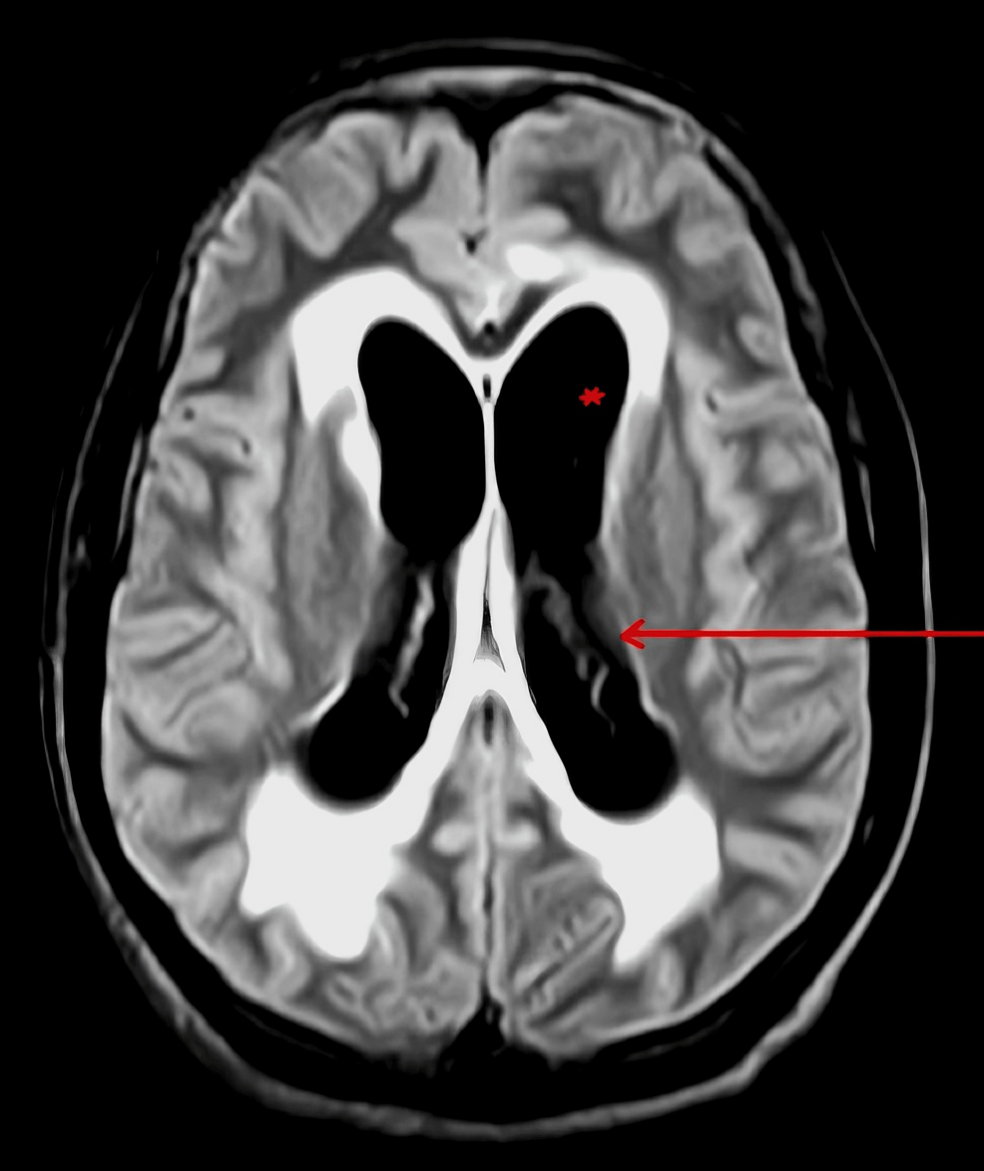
Shows MRI FLAIR image with dilated bilateral ventricle with hyperintensities in periventricular deep white matter. FLAIR- Fluid-attenuated inversion recovery
